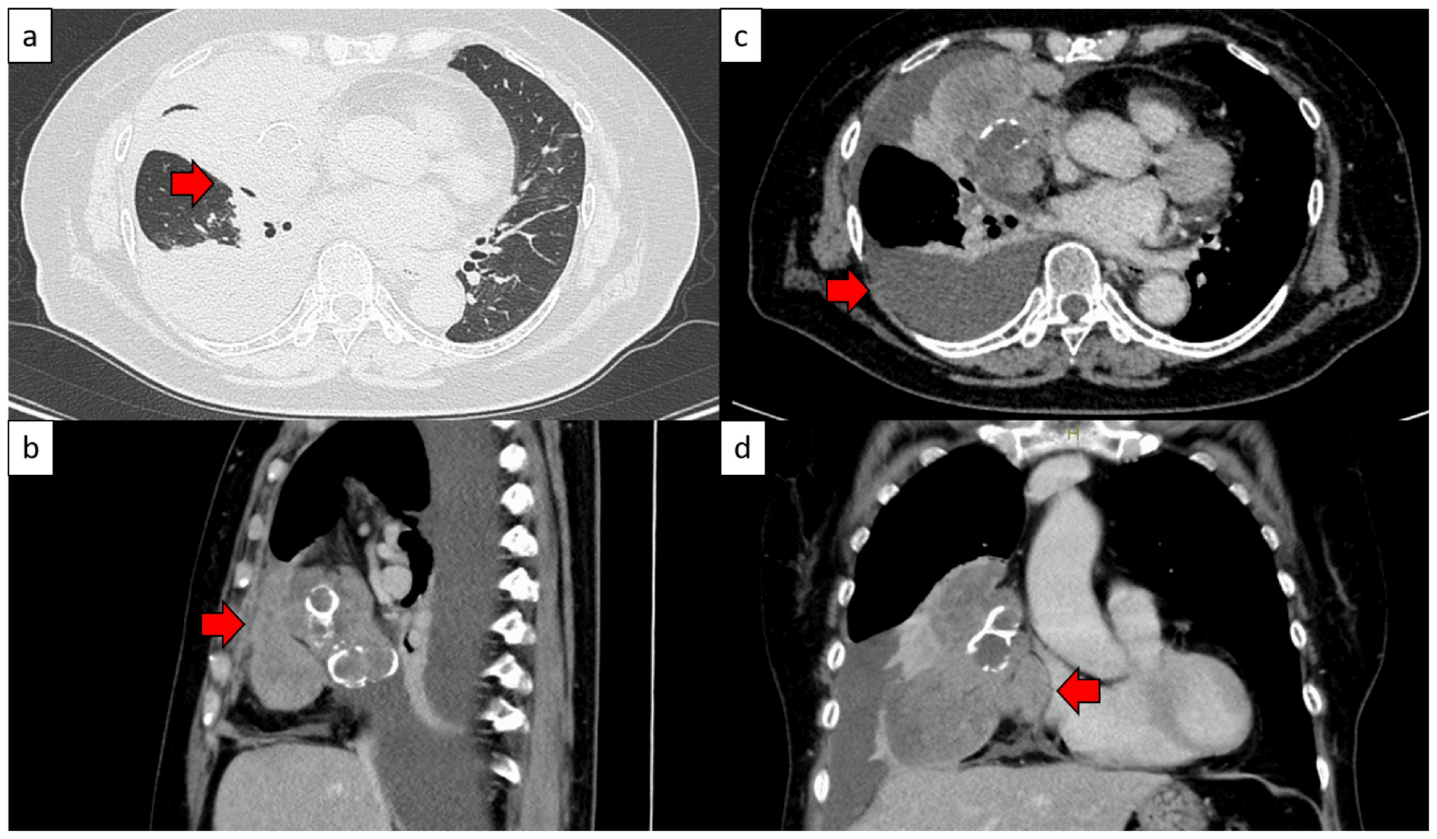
Preoperative chest computed tomography (CT) results (a) Infiltration into the right middle and lower lobes was suspected. ➡: Suspected infiltration into the right middle and lower lobes (b) Although potential right pleural infiltration was observed, apparent right chest wall infiltration was not. ➡: Right pleural infiltration (c) Right pleural effusion was observed. ➡: Right pleural effusion (d) Although suspected epicardial infiltration was observed, intrapericardial infiltration was not. ➡: Suspected epicardial infiltration
